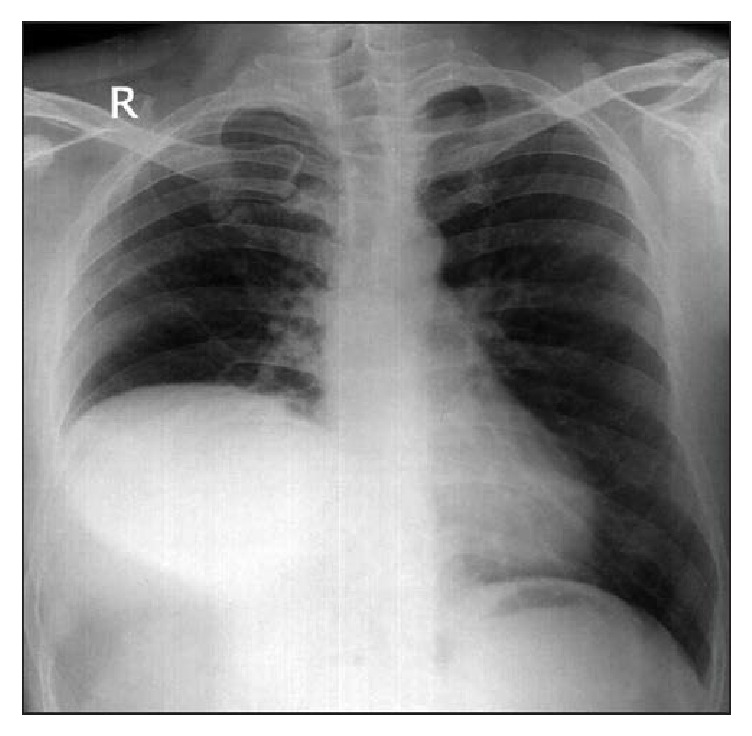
Chest X-ray with right hemidiaphragm paralysis.
